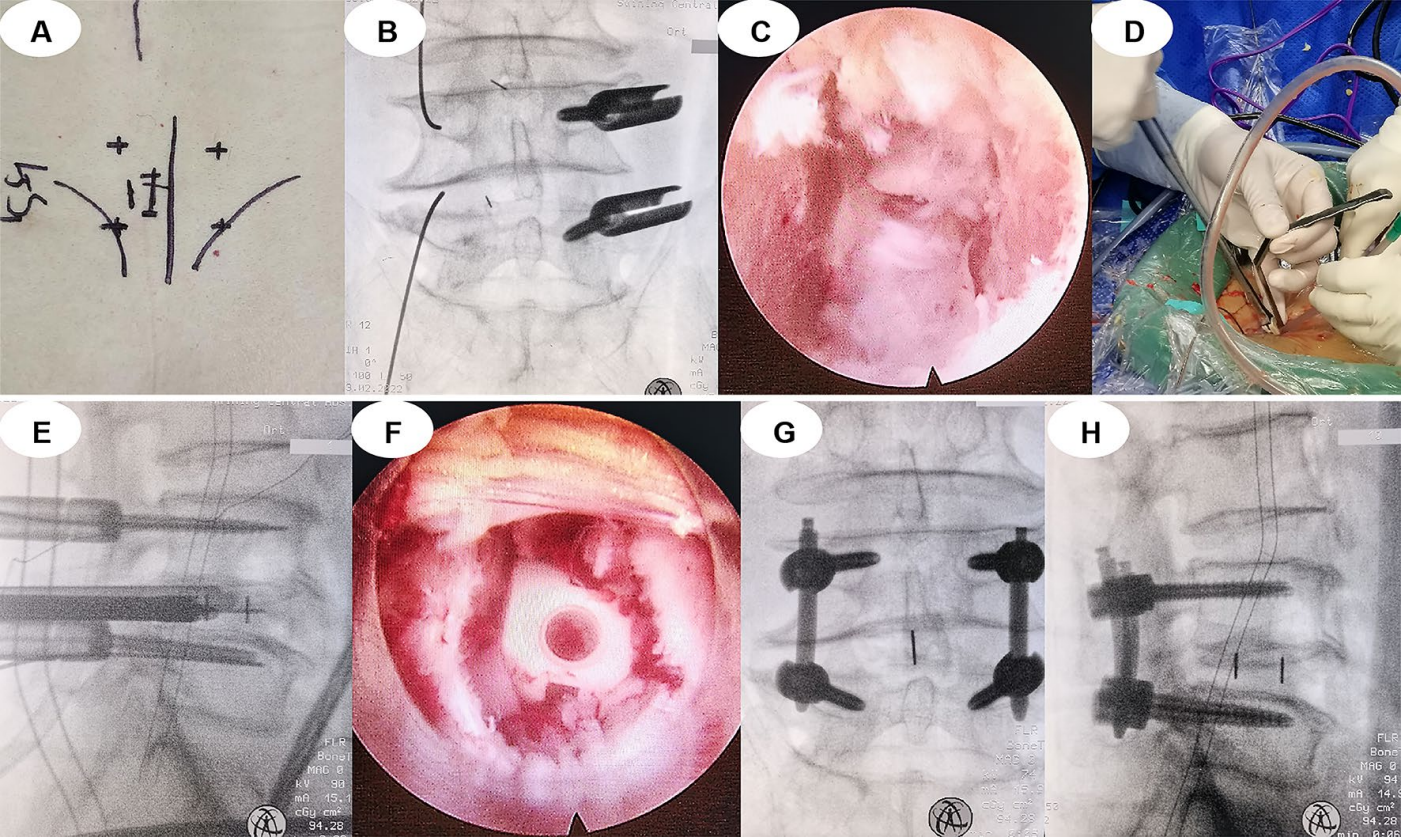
A typical Endo-TLIF case. (A) Marks of the L4/L5 vertebral pedicle and incision. (B) Posterior unilateral percutaneous pedicle screw fixation. (C) Discectomy, endplate preparation and nerve root decompression under endoscopy. (D–F) Implantation of the cage. (H) Position of cage and pedicle screws in anteroposterior and lateral X-ray views.
